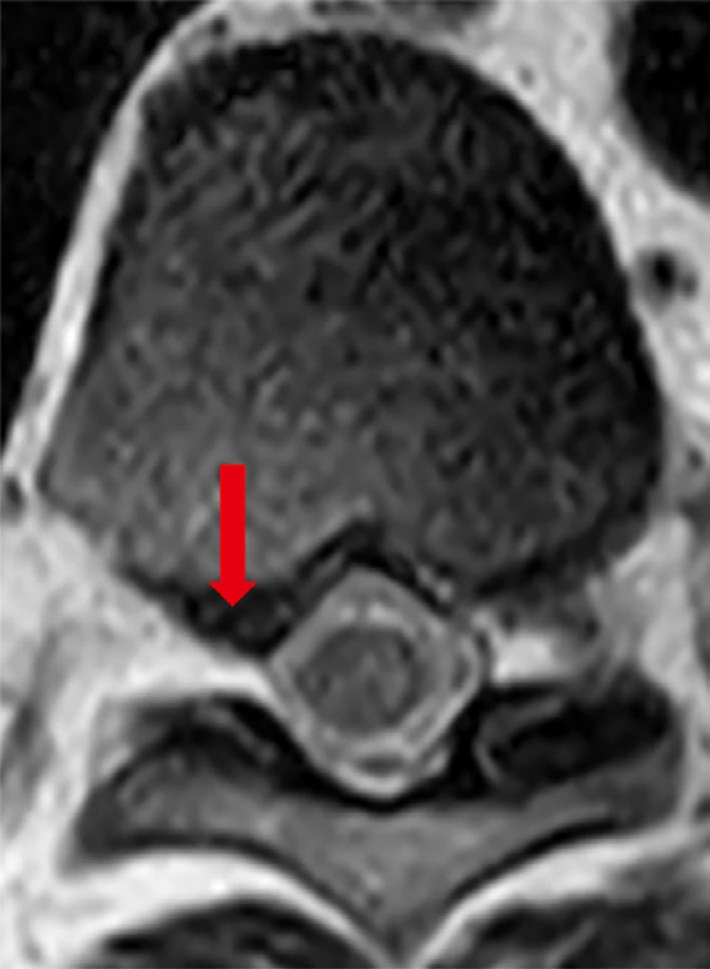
Thoracic spine MRI revealed posterior intervertebral disk protrusion in the right paramedian region at the 9th/10th thoracic vertebrae
